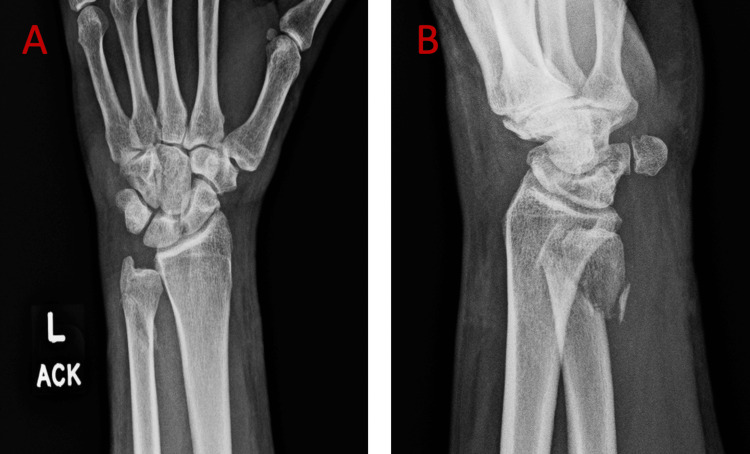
Anterior-posterior (A) and lateral (B) X-ray views of the left wrist on initial presentation revealing a minimally displaced fracture of the distal ulna.
